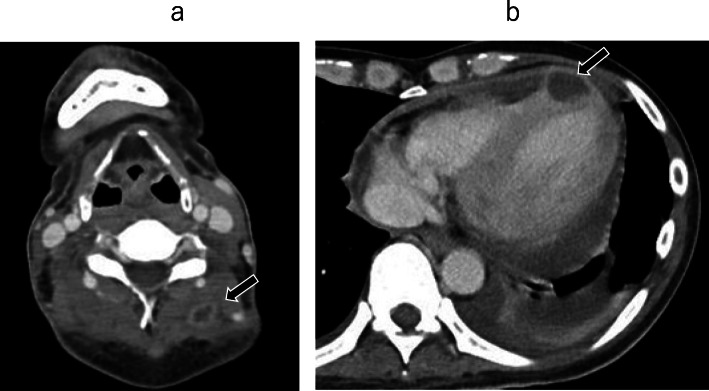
Preoperative contrast-enhanced computed tomography findings. Contrast-enhanced computed tomography revealed intramuscular low-enhancing lesions in the left posterior cervical region (a: arrow) and low-enhancing lesions in the left ventricle wall (b: arrow)
